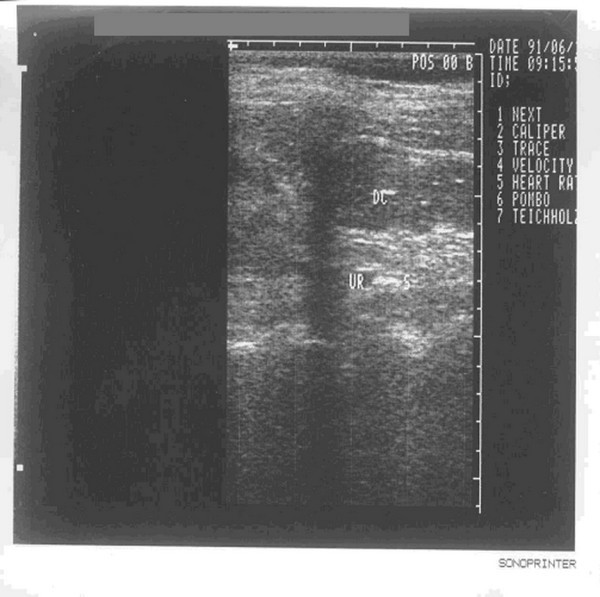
Ureter calculus. Seen under transabdominal ultrasound after normal saline retention enema, calculus (indicted by S) of the left ureter (indicted by UR) is visible behind the descending colon (indicated by DC).
